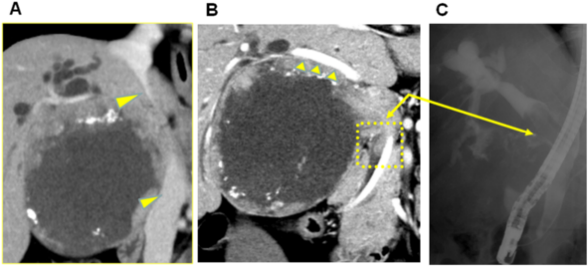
Abdominal computed tomography scans reveal a large, centrally necrotizing and well-demarcated tumor. (A) The tumor compressed the neighboring tissue Yellow arrowheads indicates the inferior vena cava. (B) Yellow arrowheads indicate the right intrahepatic Glisson. (C) Endoscopic retrograde cholangiopancreatography reveals that the tumor extended to the intrapancreatic bile duct (arrows in B and C).
